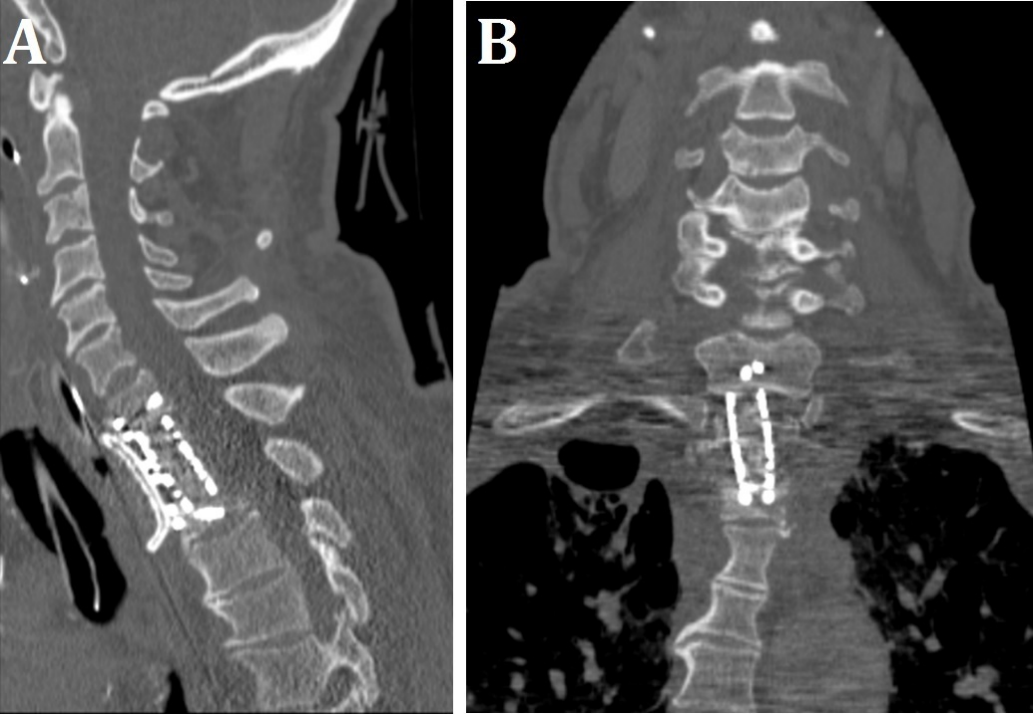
Postoperative CT Imaging Postoperative (A) sagittal and (B) coronal CT imaging showing interval partial corpectomy of T1 and T2 vertebral bodies with anterior spinal fusion extending from C7 through T2 vertebral bodies.
